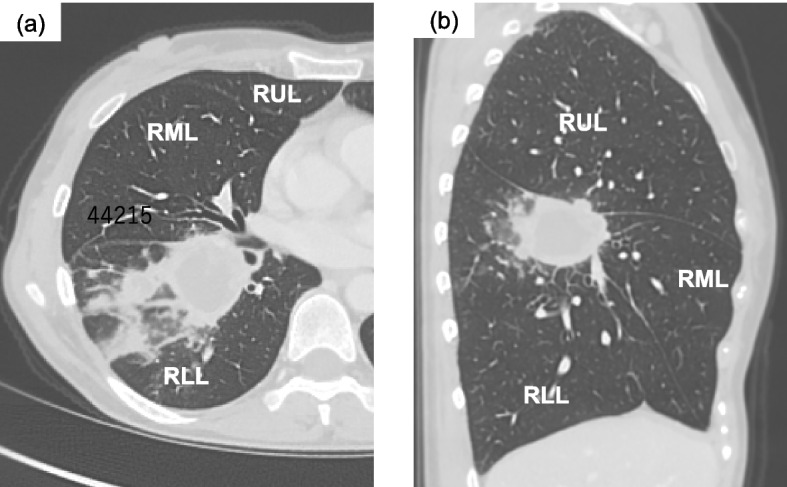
Preoperative chest computed tomography (CT). a Axial CT image showing a lung mass predominantly involving the right lower lobe with extension toward the middle lobe. b Sagittal reconstruction showing the mass centered in the superior segment (S6) and located at the confluence of the three lobes
